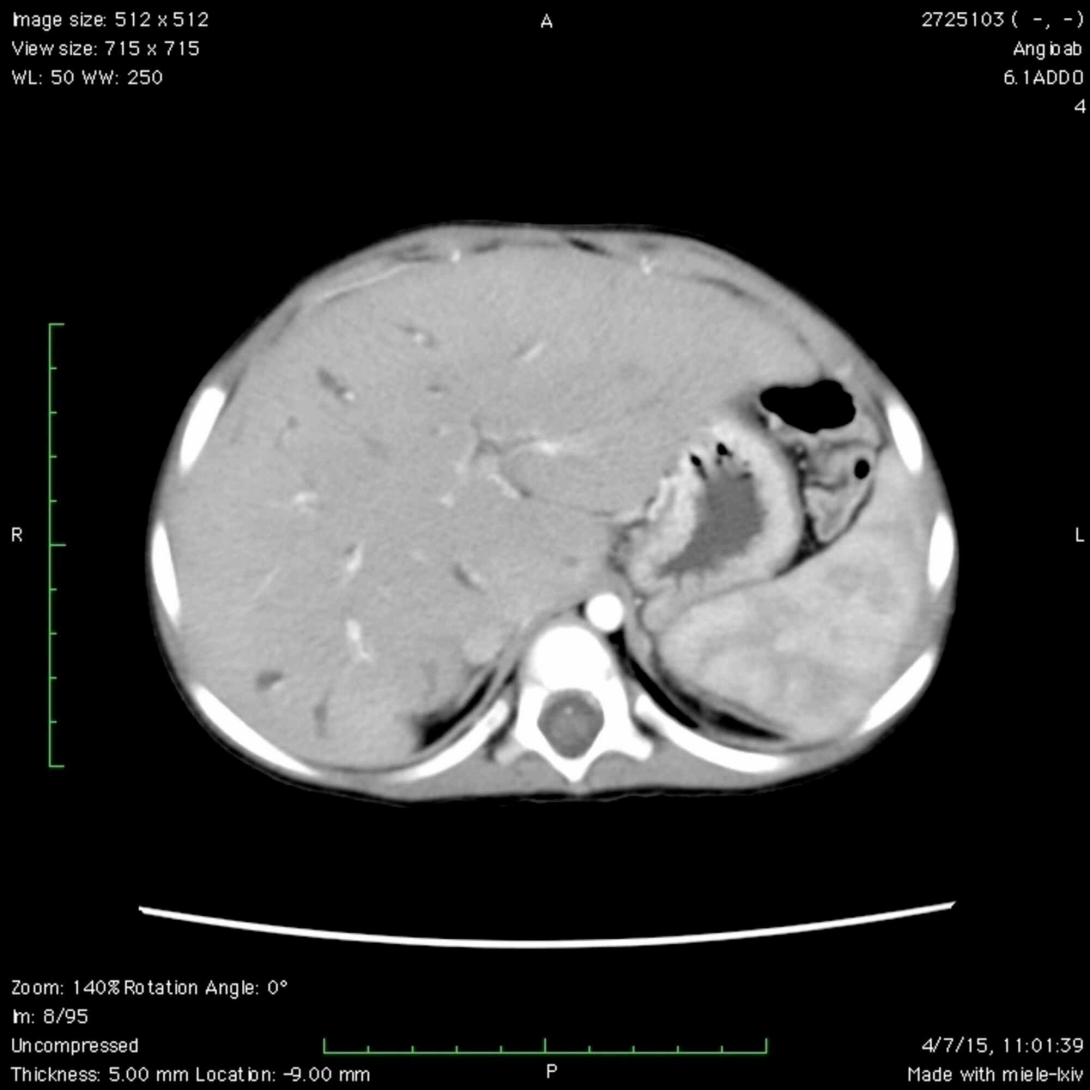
Computed tomography showing dilated saccular bile ducts
